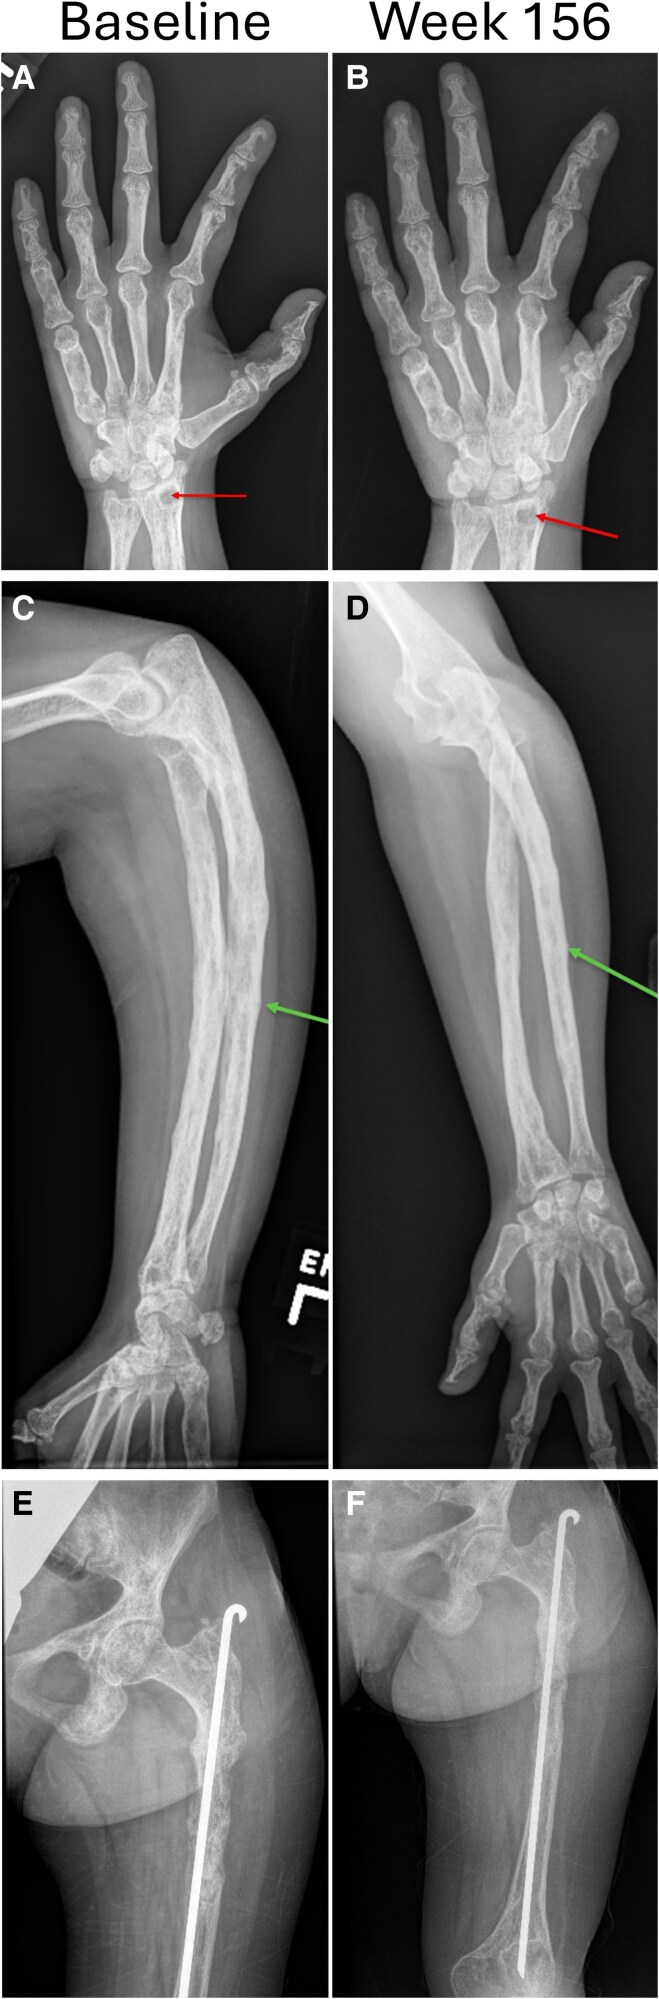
Radiographs at baseline and after 156 weeks of burosumab of the A and B, left hand/wrist; C and D, forearm; and E and F, pelvis/femur. At baseline there was noticeable bony heterogeneity with areas of sclerosis and bone resorption; intramedullary and cortical cysts are present (arrow). With burosumab treatment, bone mineralization improved with reduction in cortical irregularity and resolution of the ulnar fracture (arrow). The cortical cyst remained unchanged.
